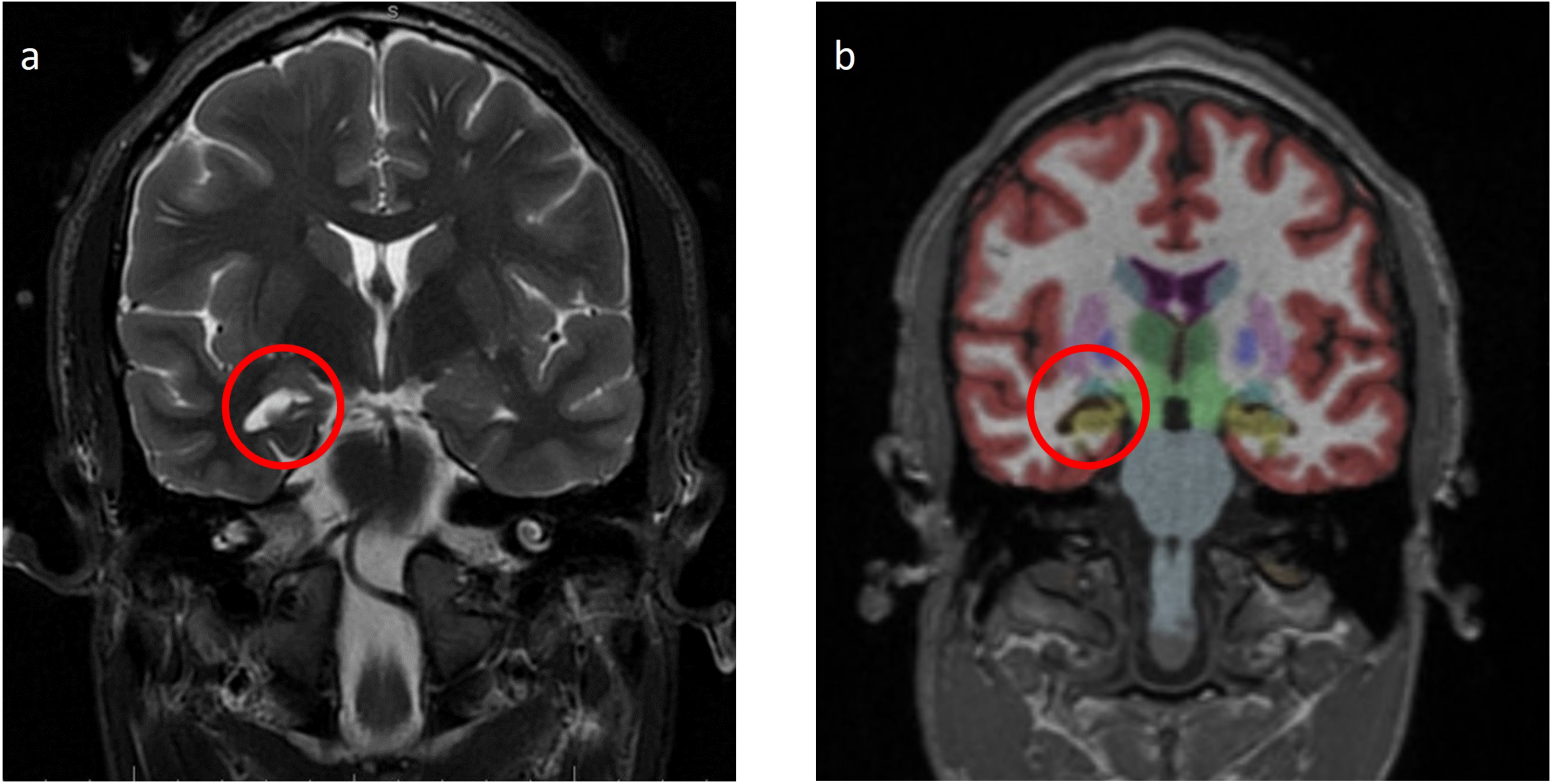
(A) Magnetic resonance imaging (MRI) Brain W/WO Contrast T2 Fluid Attenuated Inversion Recovery (FLAIR): low right hippocampus volume (red circle). (B) MRI Brain with NeuroQuant a few weeks after the initial admission shows the low right hippocampus volume (fifth percentile, yellow area in the red circle).
